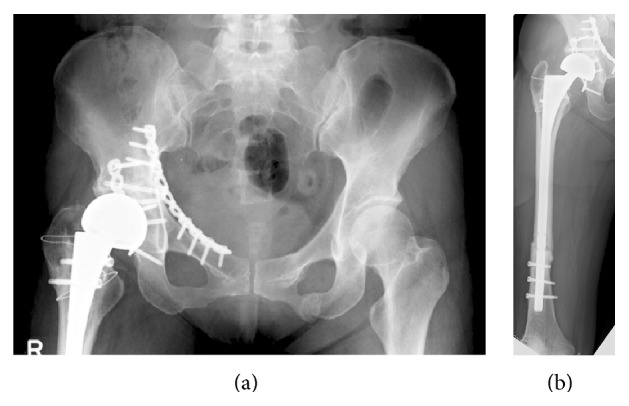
Anteroposterior radiographs of the pelvis (a) and femur (b) 1 year after arthroplasty.
